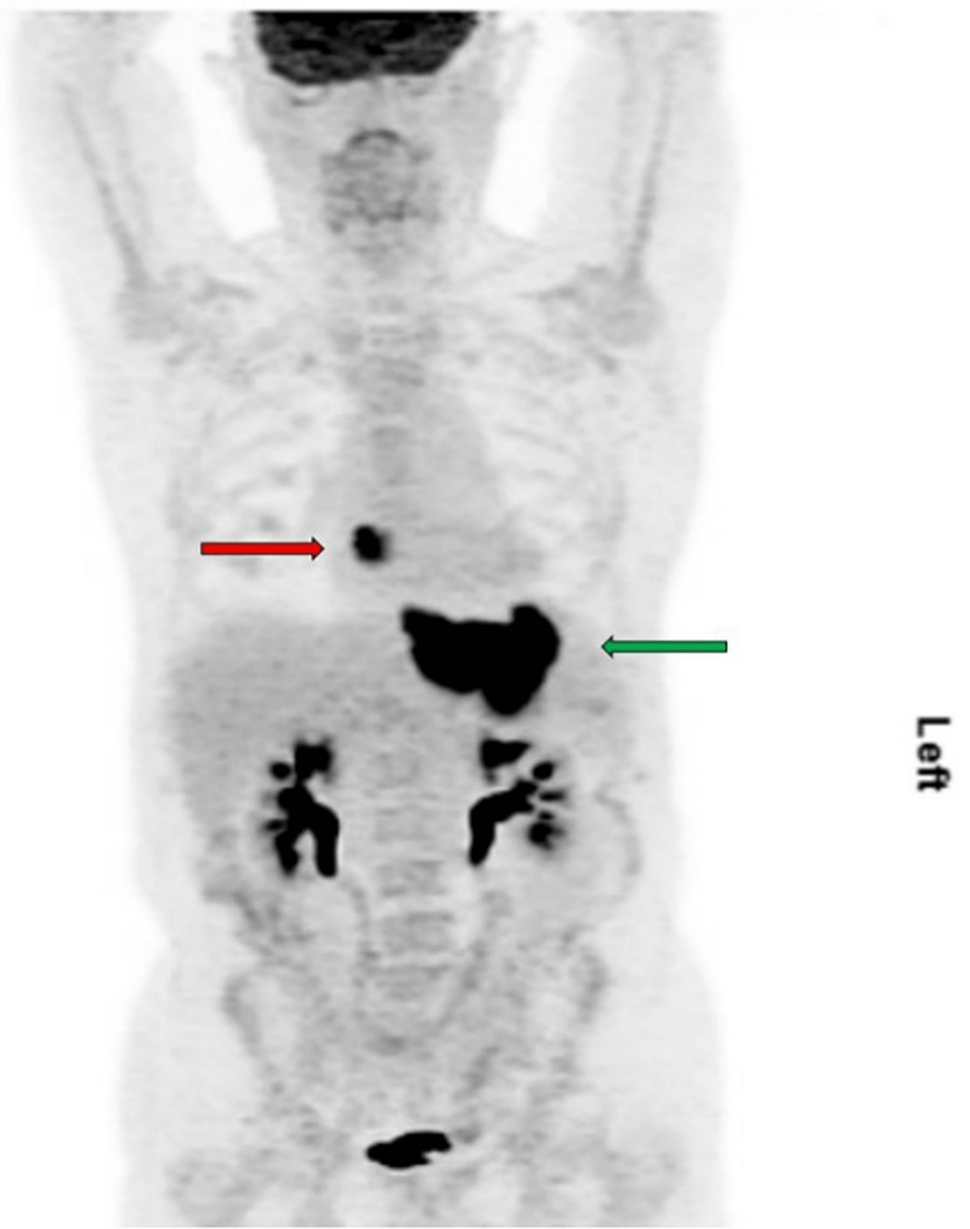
F18-FDG PET image showing left atrial mass (red arrow) and primary tumor (green arrow). F18-FDG PET, fluorine-18 fluorodeoxyglucose positron emission tomography
